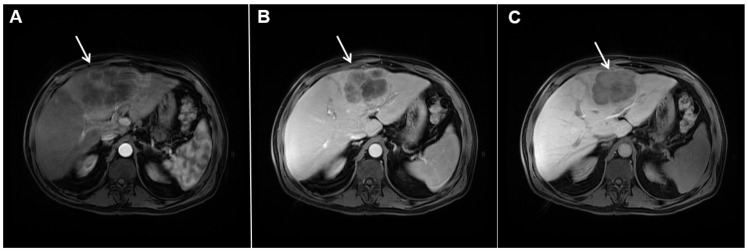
Mass-forming intrahepatic cholangiocarcinoma in a 68-year-old woman. Axial T1-weighted image after gadoxetic acid administration obtained in arterial phase (A) shows peripherally enhancing lesion (*arrow*). Portal venous phase in the same patient (B) shows progressive centripetal enhancement of the lesion (*arrow*) with cloud-like appearance in the hepatobiliary phase (C) consisting of an area of central enhancement and a thin, peripheral, hypointense rim.
